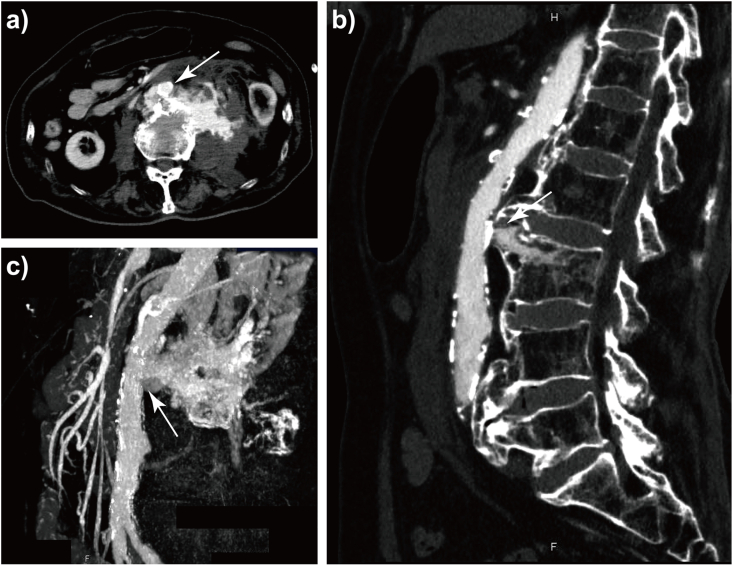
Enhanced-CT and angiography. (a) (b) The compression fracture of the lumbar vertebra (L3), with sharp osteophyte (white arrow), injured the abdominal aorta (c) Contrast media was spurting from the abdominal aorta (white arrow) into the retroperitoneum.
